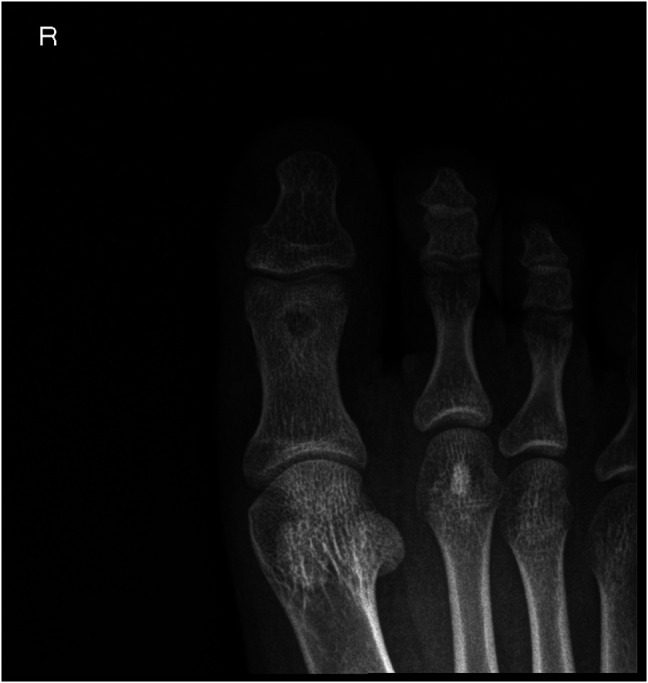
Follow-up radiograph obtained at 1 year after the surgical excision revealed no signs of recurrence in the proximal phalanx of the great toe.
